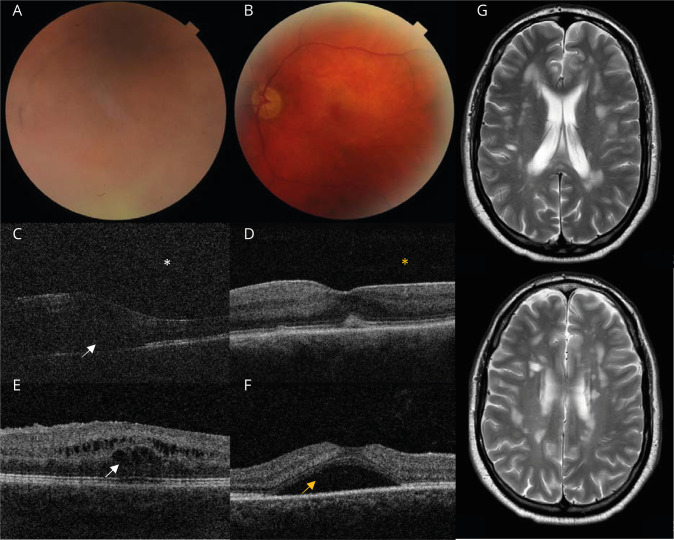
Fundal changes associated with intermediate uveitis and the complications of treatment Fundus photographs (A and B) and corresponding OCT images (C and D) taken from the same patient before (A and C) and after (B and D) treatment for IU. (A and C) Pretreatment images show how severe active vitritis makes the fundal view hazy (A) and blur OCT images of the retina (C). The white asterisk highlights the appearance of vitritis on OCT, which looks like a gray snowstorm. The white arrow points to coincident neuroretinitis causing retinal thickening in the same patient. (B and D) Posttreatment images show how the resolution of active vitritis mean that the fundal view (B) and OCT image of the retina (D) become clearer and the vitreous appears black (yellow asterisk). (E) OCT image showing cystoid macular edema: a complication of IU and fingolimod. The white arrow points to intraretinal cystic spaces and retinal thickening. (F) OCT image of central serous chorioretinopathy: a complication of steroid treatment. The yellow arrow points to subretinal fluid. (G) Two MRI brain slices from the same patient who developed demyelination following exposure to anti-TNF biologic therapy, demonstrating high T2 signal in the periventricular and deep white matter. Images are supplied courtesy of Dr. C. Rice, Dr. L. Kobayter, and Mr. T. Burke. IU = intermediate uveitis; OCT = optical coherence tomography.
